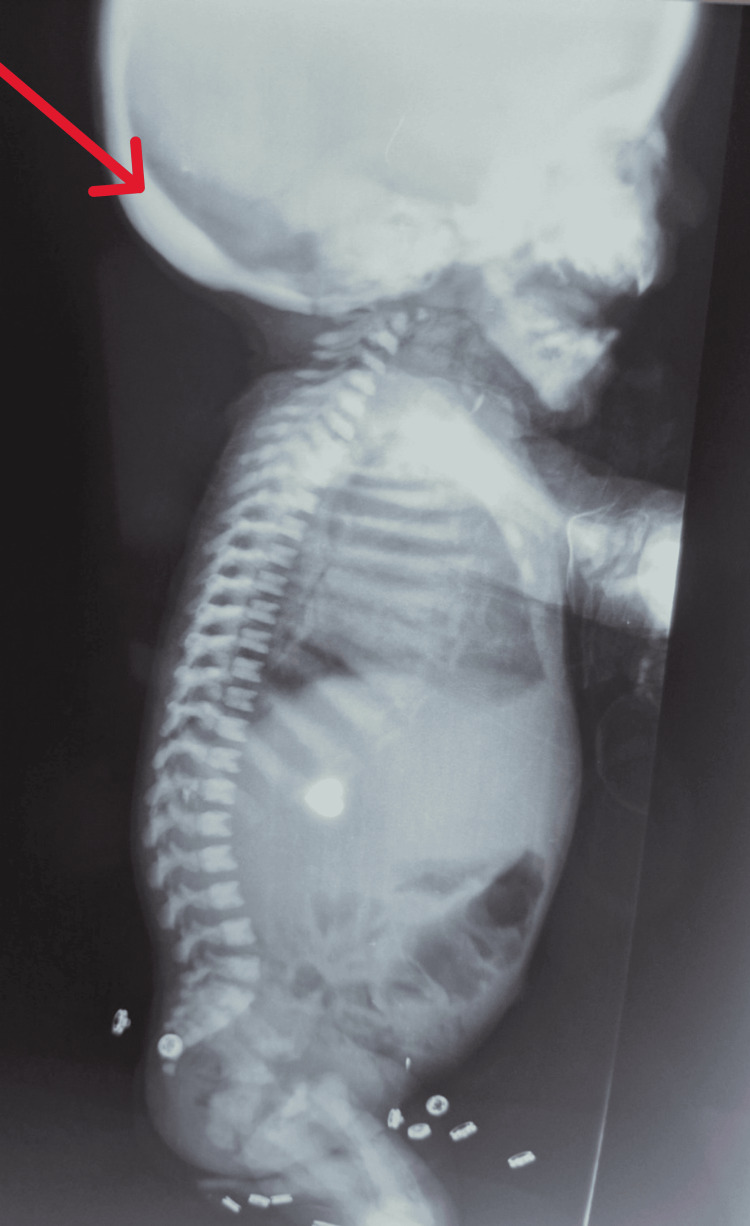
Lateral radiograph of the skull and spine showing pronounced calvarial thickening and homogeneous hyperdensity Lateral radiograph of the skull demonstrating pronounced calvarial thickening and homogeneous hyperdensity with narrowing of the cranial foramina, consistent with malignant infantile osteopetrosis. The arrow indicates the area of calvarial thickening.
